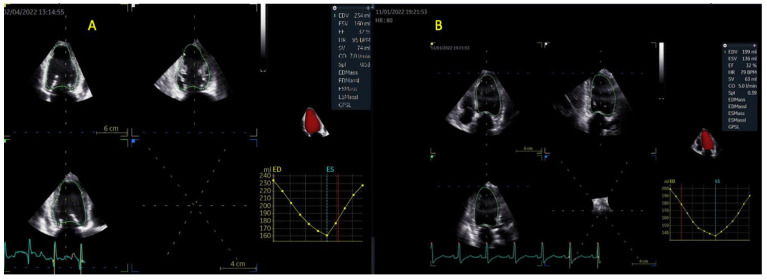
LV volumes and EF calculation via 3DE in a patient with (A) AF or (B) SR. Abbreviations: LV—left ventricular; EF—ejection fraction; 3DE—three-dimensional echocardiography; SR, AF—same as in Figure 1.
